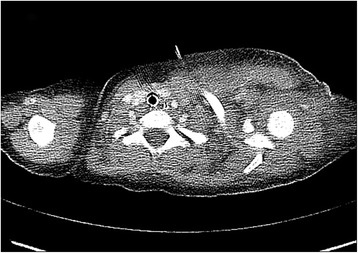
Computed tomography shows diffuse swelling of the lateral chest wall and axilla 4 days after the initial surgery.
